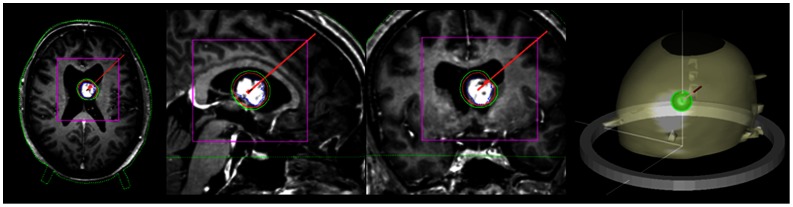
Precise treatment planning on axial, sagittal and coronal T_1_-weighted, gadolinium enhanced MR images (blue dotted line: tumor border; red line: 65 Gy isodose; green line: 50 Gy isodose) and 3D-trajectory plan (figure 1d).
